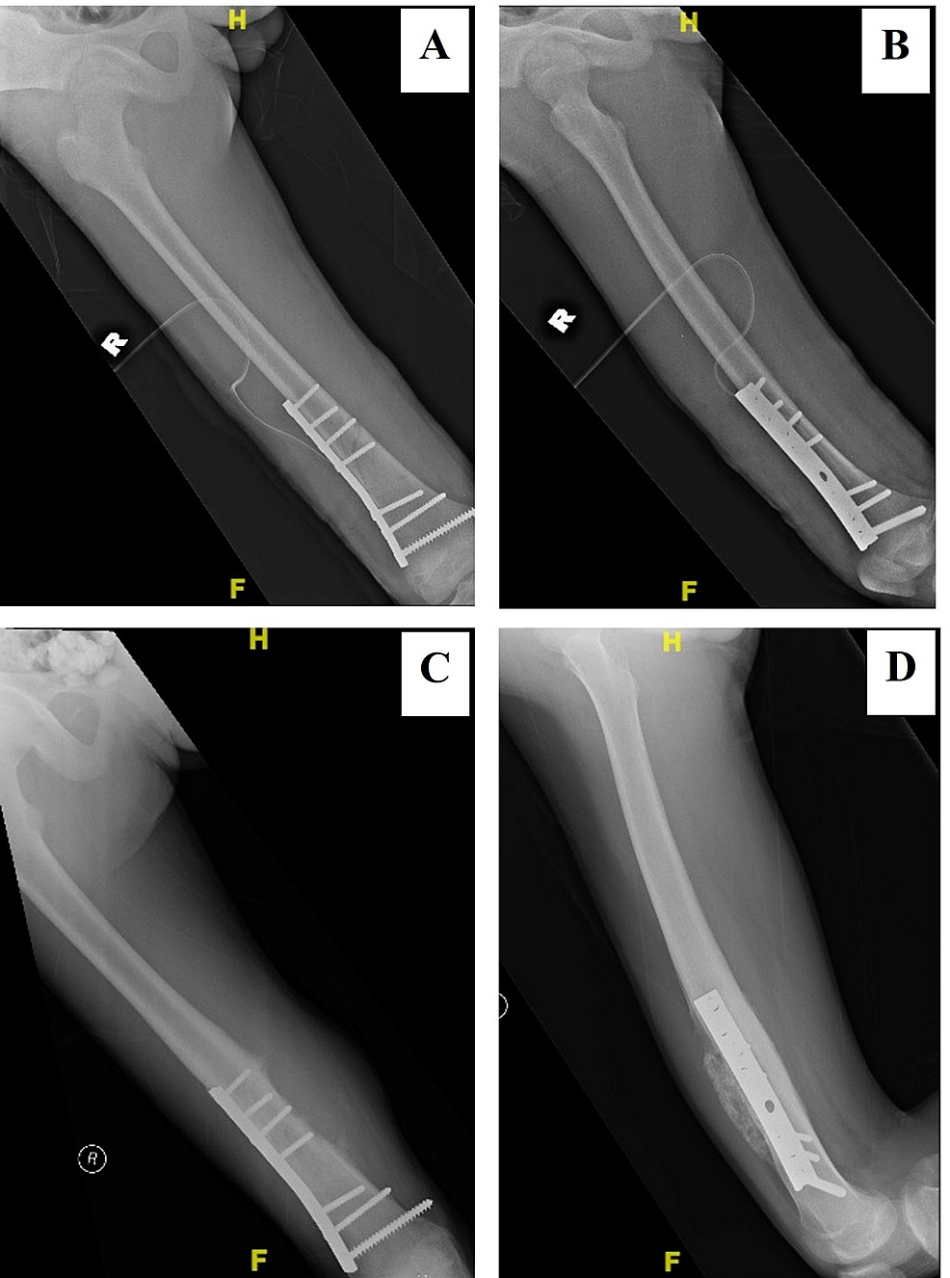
A distal third right femoral fracture stabilized with a narrow dynamic compression plate The initial plain radiographs did not show any evidence of malignancy (A, B). Three years after the surgery, periosteal reaction with the Codman triangle was seen at the medial aspect of the united fracture (C) with calcification of its surrounding soft tissue at the anterior aspect (D). H: head or cranial/ top part of the limb, R: right, F: foot or caudal/ lower part of the limb.
